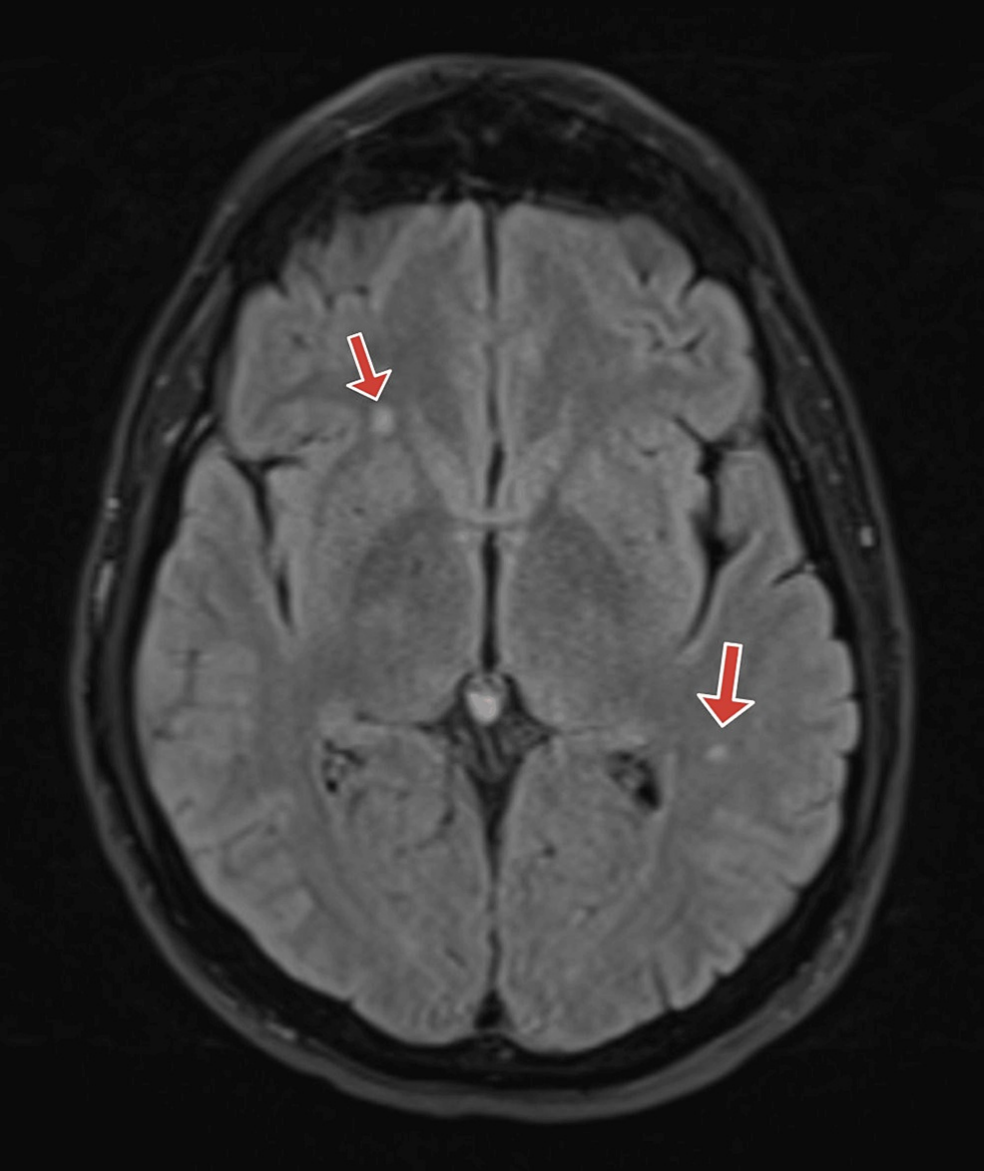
Brain MRI Brain MRI with red arrows pointing at bilateral white matter changes thought to be related to an infection or an inflammation.
